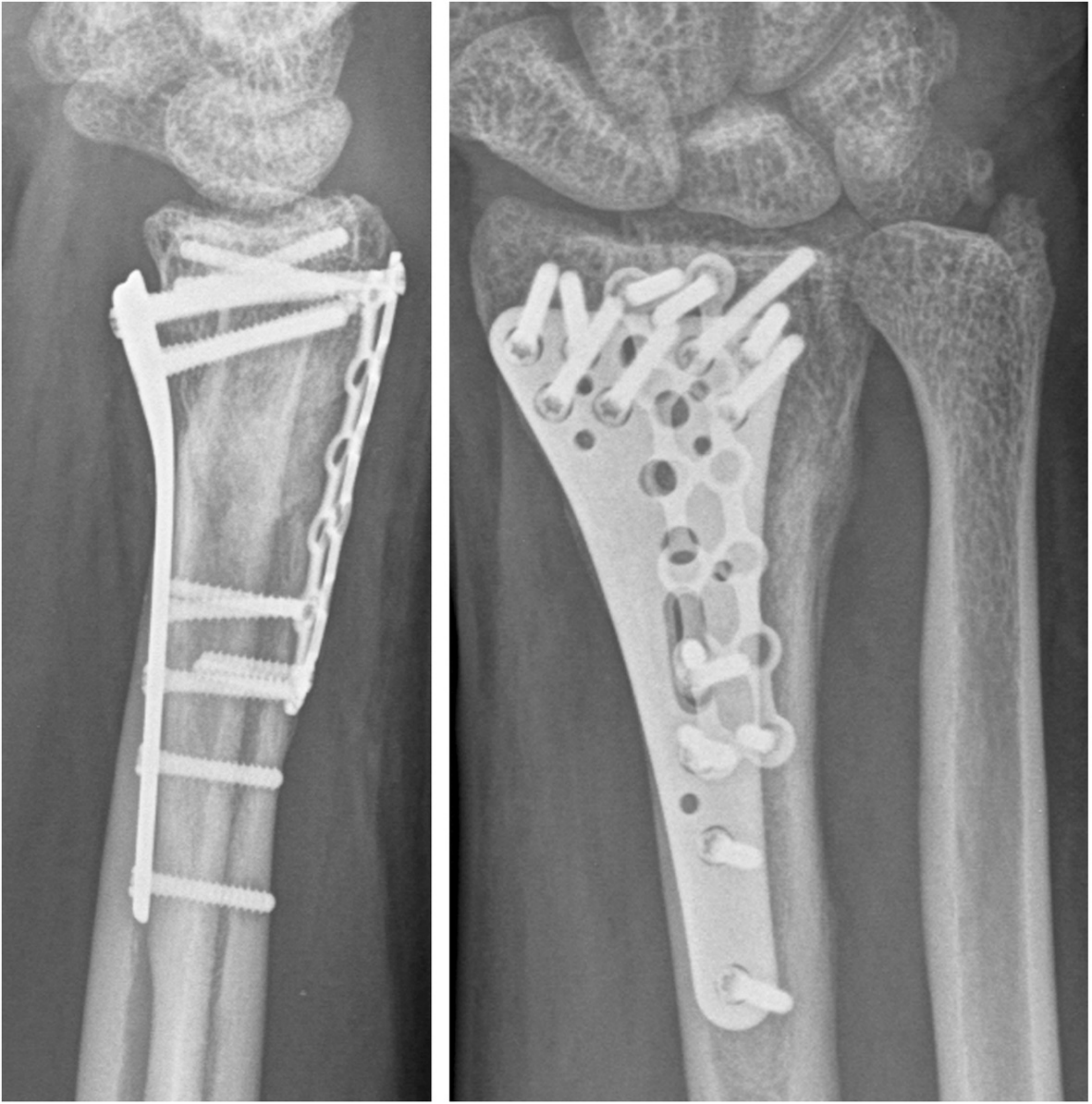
Radiographs at final follow up 6 months postoperative. The images show a well reconstructed and anatomically united distal radius.
